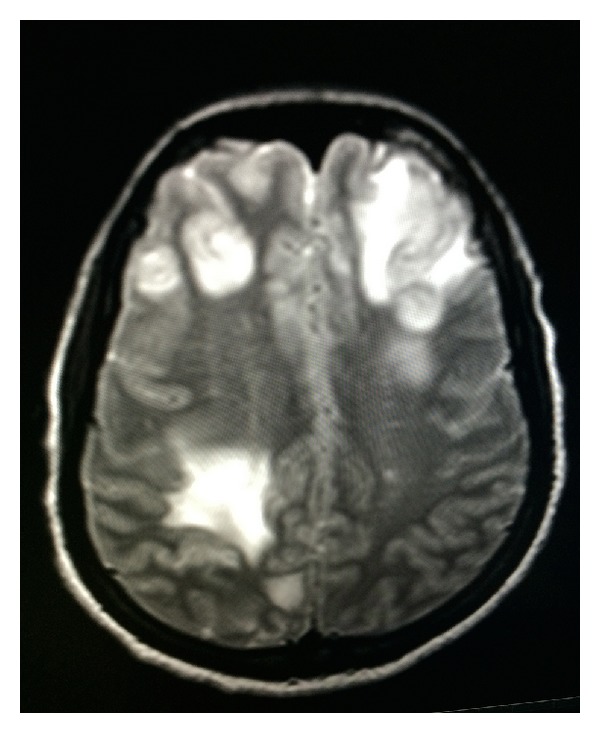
MRI-T2-Axial View: left frontal and right parietal.
